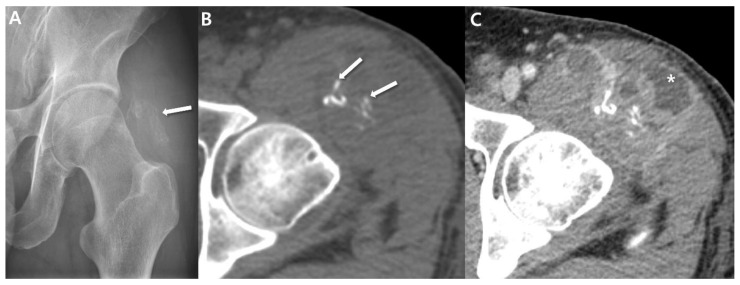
CT features of synovial sarcoma of 54-year-old man (undifferentiated type) containing coarse calcifications: (A) Pelvis outlet radiograph shows coarse calcifications (arrow) in soft tissue opacity of Lt upper thigh. (B) Axial precontrast CT shows coarse calcifications (arrows) in large mass with heterogenous in density. (C) Axial post-contrast CT shows heterogeneously enhancing mass including non-enhancing region (*), suggesting necrotic or cystic change.
